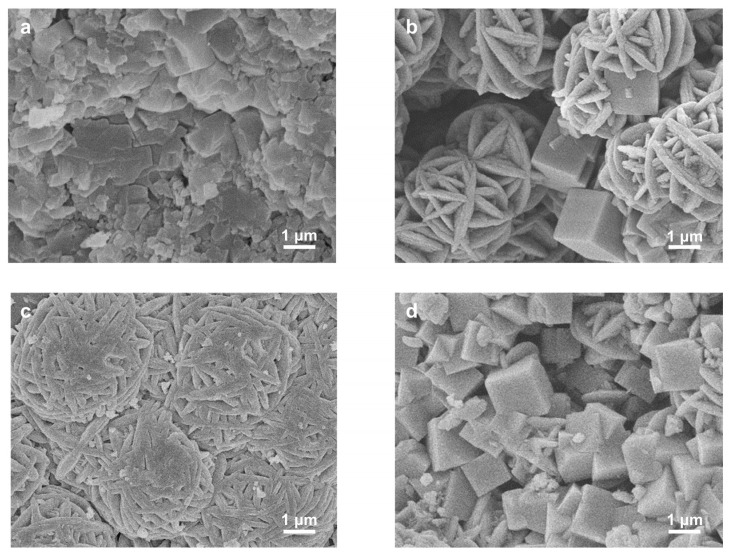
Controlling factors for generating spherical NaA-500 with spatially uniform crystal assembly: (a,b) Effect of vacuum infiltration during silica impregnation showing non-uniform zeolite microstructure obtained without vacuum infiltration of (a) internal and (b) external surface; (c,d) Effect of ultrasound pretreatment during silica impregnation on the zeolite microstructure showing expedited crystallization leading to SOD formation at 8 h.
